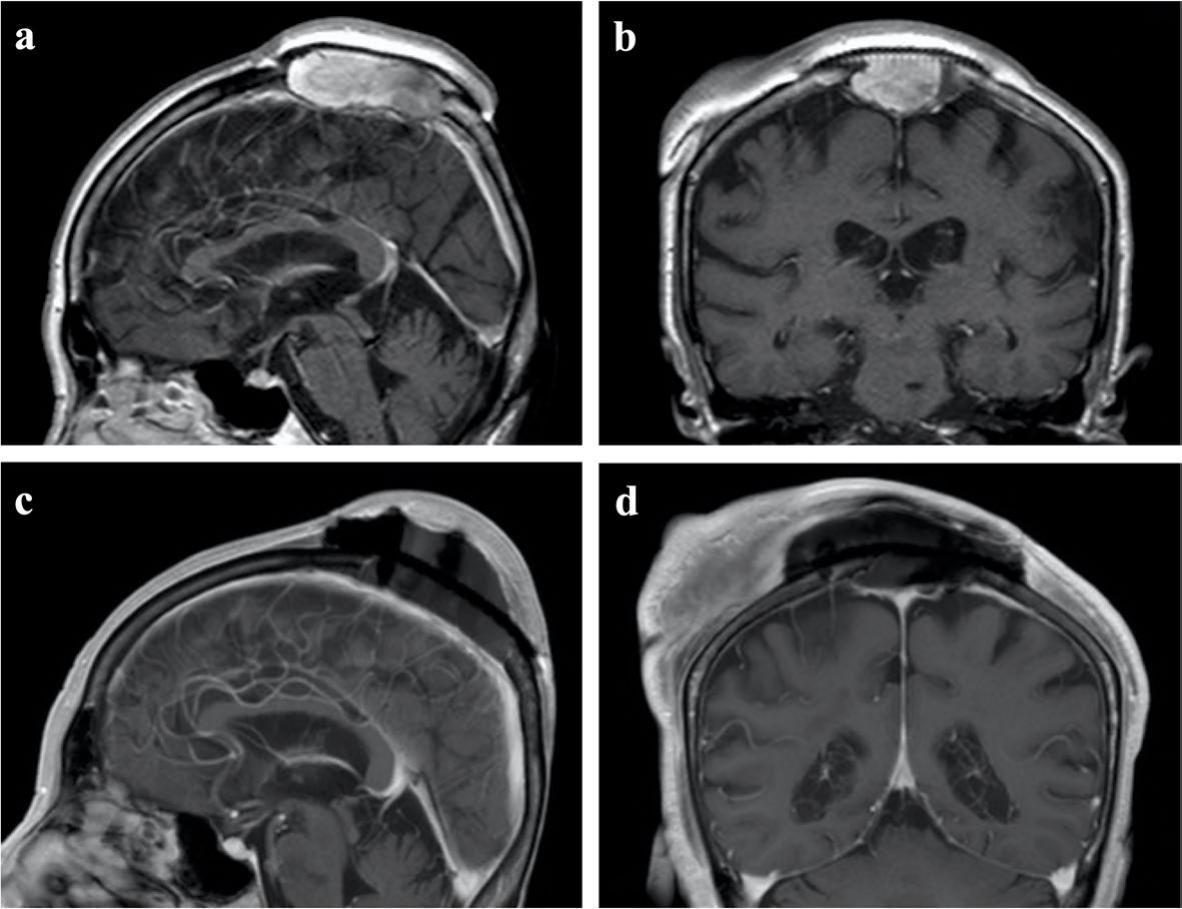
Magnetic resonance imaging before and after second surgery. Recurrence of solid tumor is seen at the superior sagittal sinus (a sagittal view; b coronal view). The tumor is totally excised, preserving the SSS, adjacent dura and pedicled scalp flap (c sagittal view; d coronal view)
